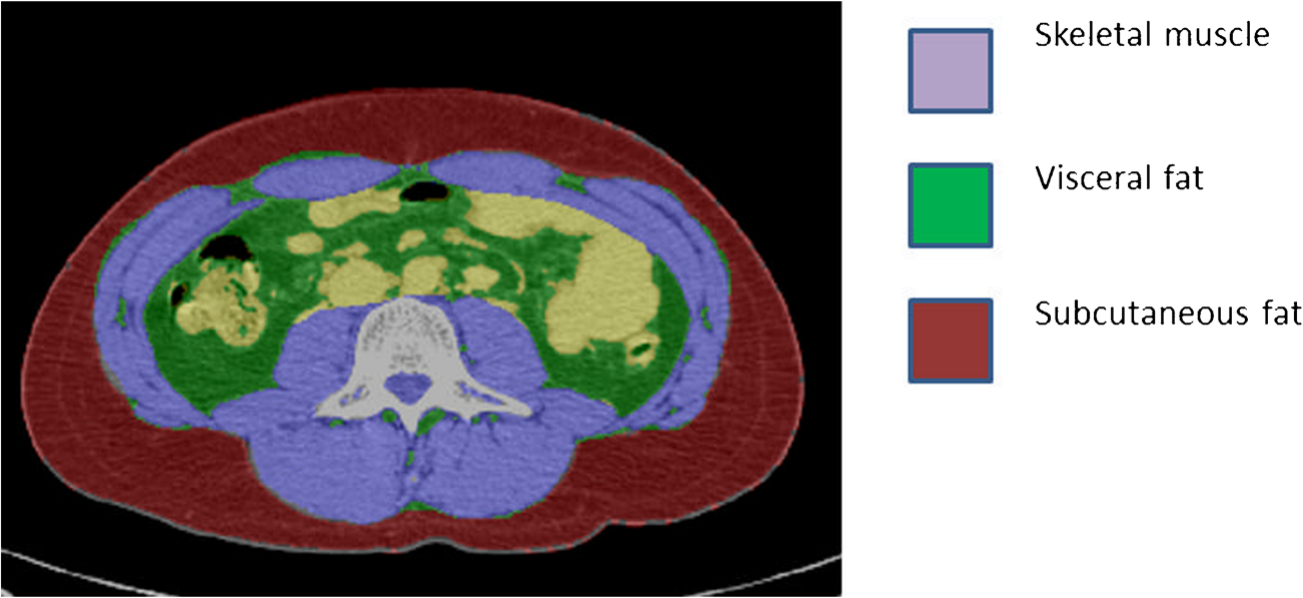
Subcutaneous fat, visceral fat and skeletal muscle as depicted on an axial CT image at the level of L3 vertebral body
